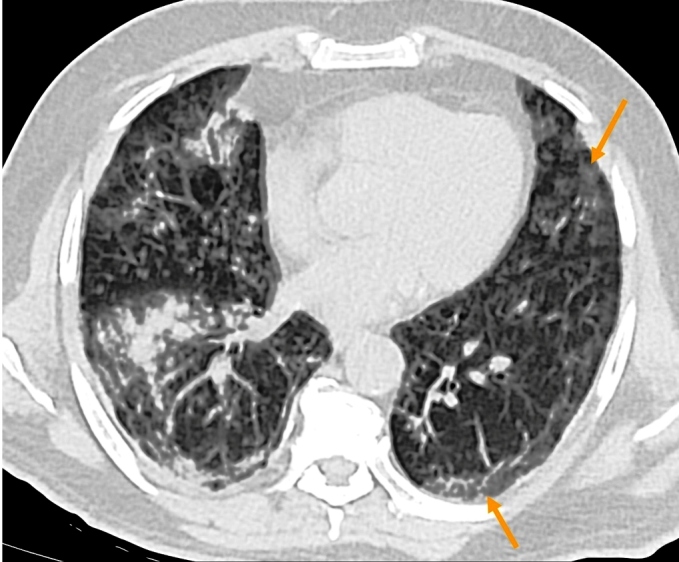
An axial slice chest CT in lung window shows multiple centrilobular
